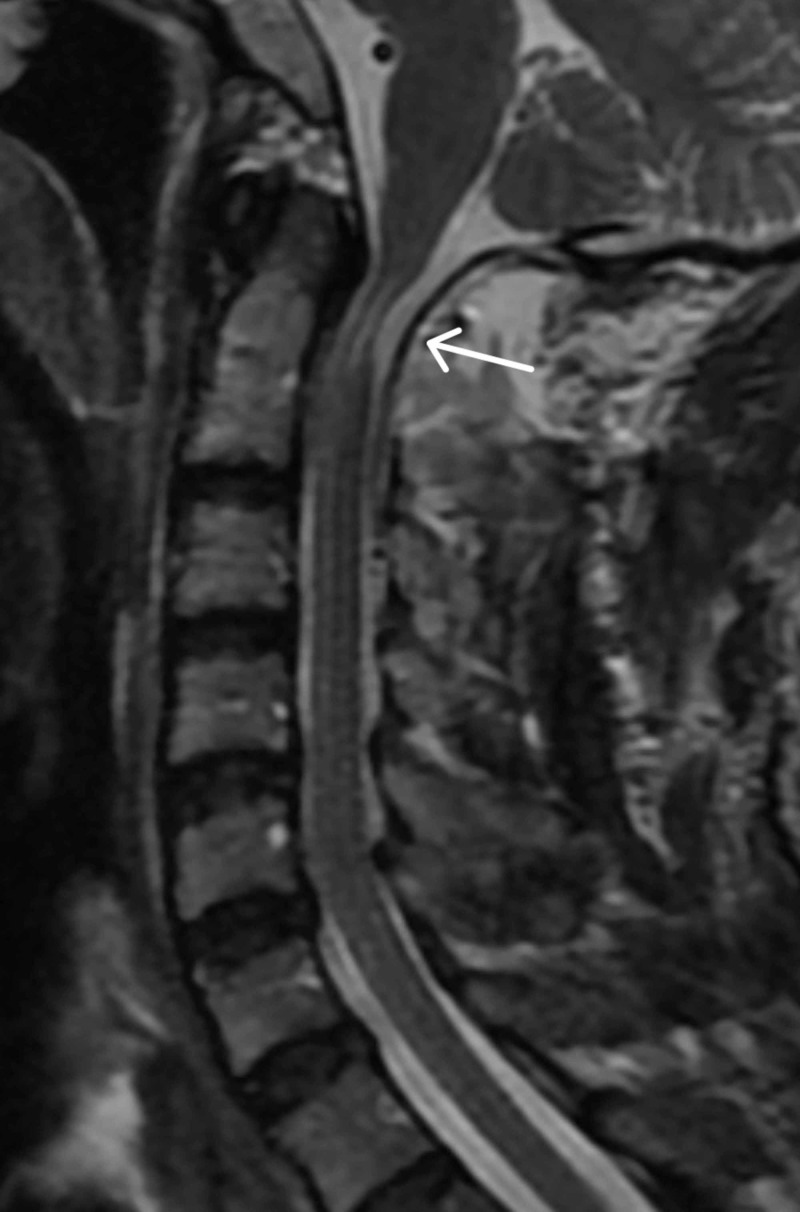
Postoperative T2-weighted MRI of the cervical spine in patient with severe cervical stenosis and cervical myelopathy Postoperative MRI showing complete decompression of cervical spinal cord and hyperintensity at C1.
